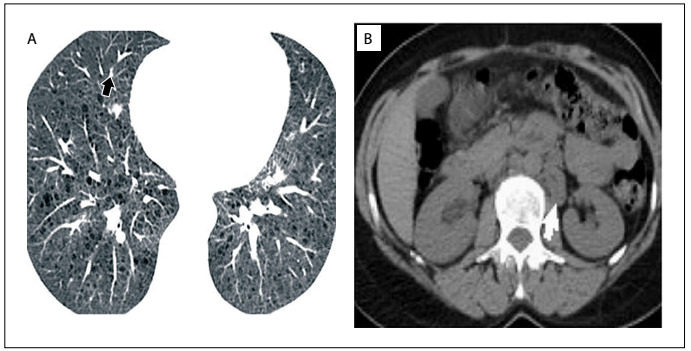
A) Axial abdominal computed tomography image showing residual tumor (arrow). B) Lung computed tomography image showing tiny diffuse parenchymal cystic air spaces.
